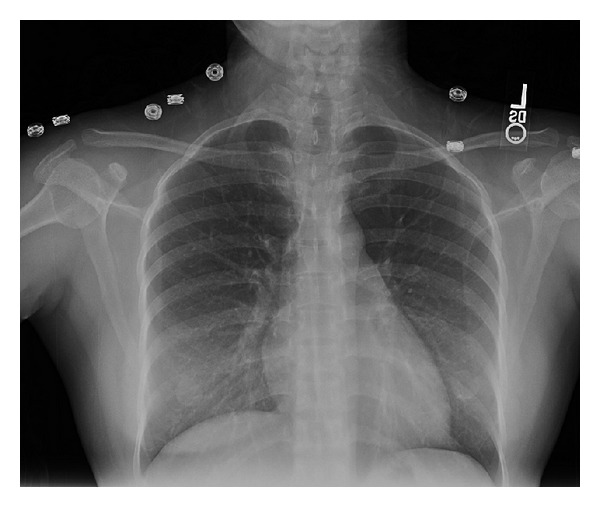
Chest X-ray showing resolution of pulmonary congestion 3 days after admission.
